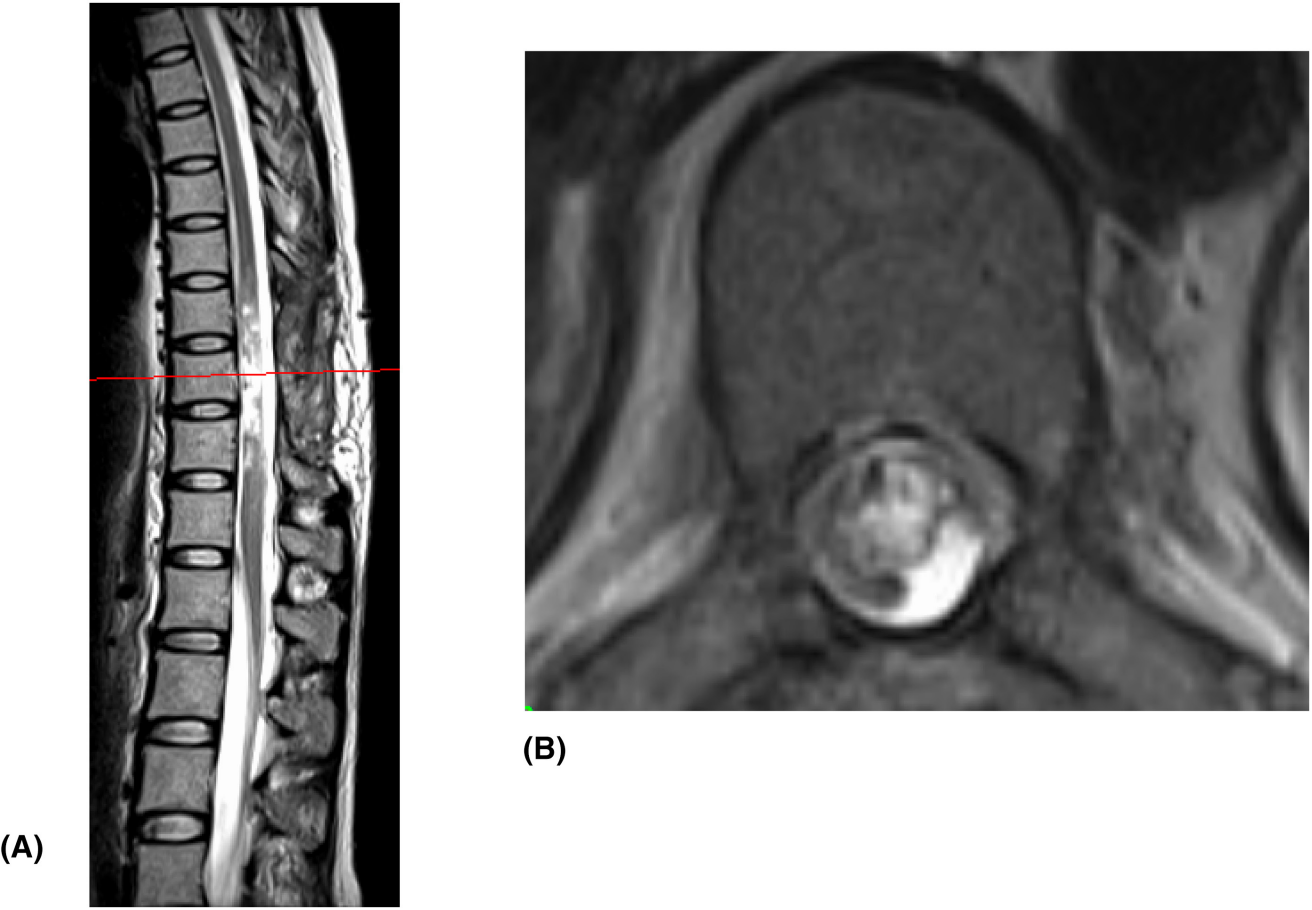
Magnetic resonance imaging (MRI) at 3 weeks after surgery. (A) Sagittal and axial. (B) T2‐weighted MRI showing resolution of the hematoma, but there remains an irregular, well‐defined hyperintense signal in the T9‐11 levels.
